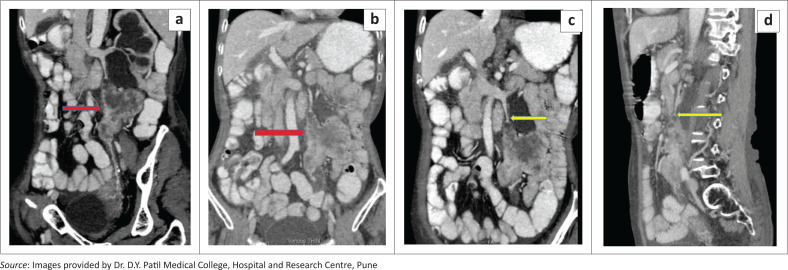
Coronal oblique (a), showing a well-defined heterogeneous mass (red arrow) along the course of left gonadal vein causing hydronephrosis and hydroureter. Coronal (b–c) and sagittal (d) reformatted CT abdomen reveal a well defined heterogeneously enhancing retroperitoneal mass (red arrow) along the course of the left gonadal vein causing filling defect (yellow arrow)(c–d).
